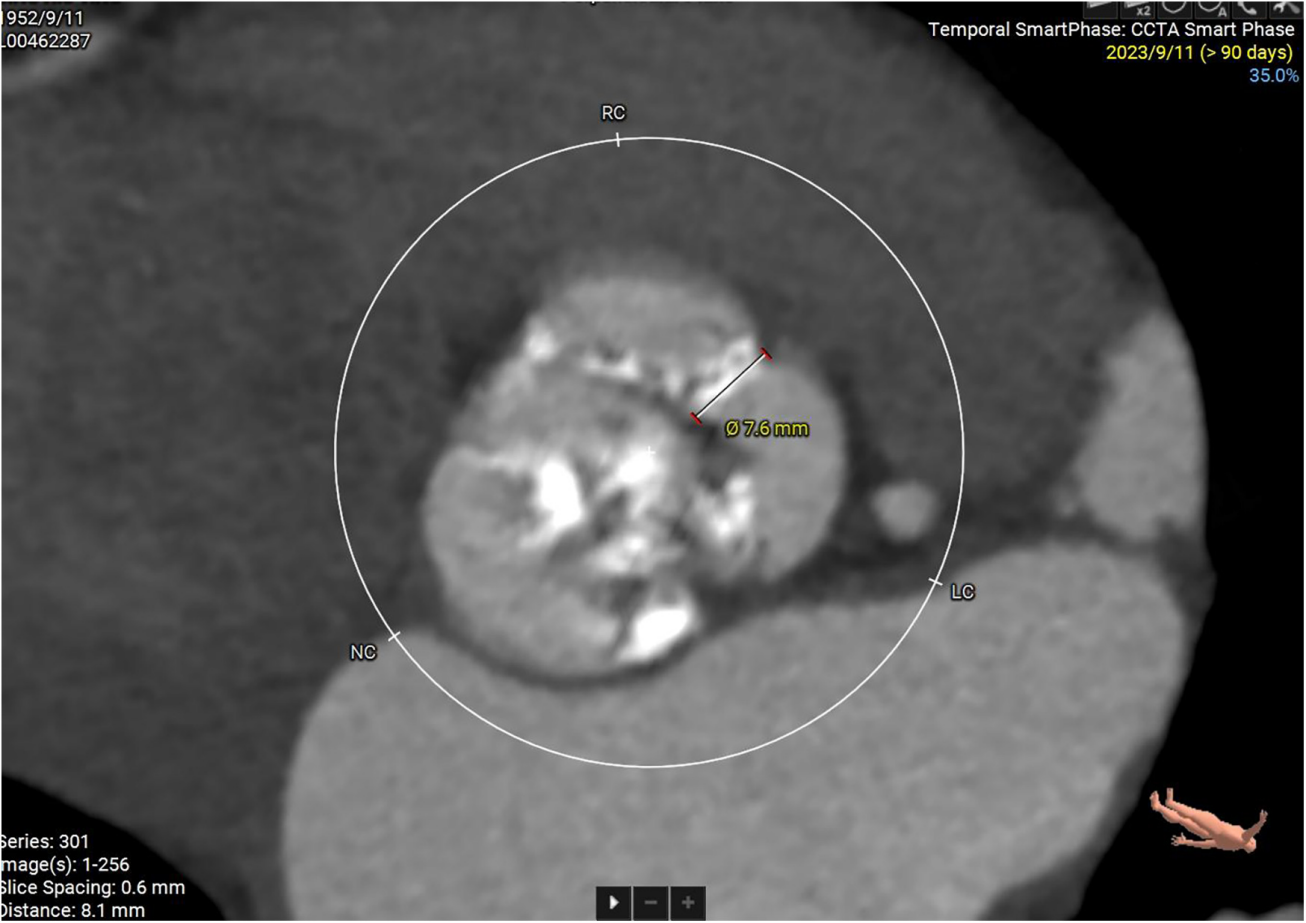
Enhanced CT examination of valve characteristics.
